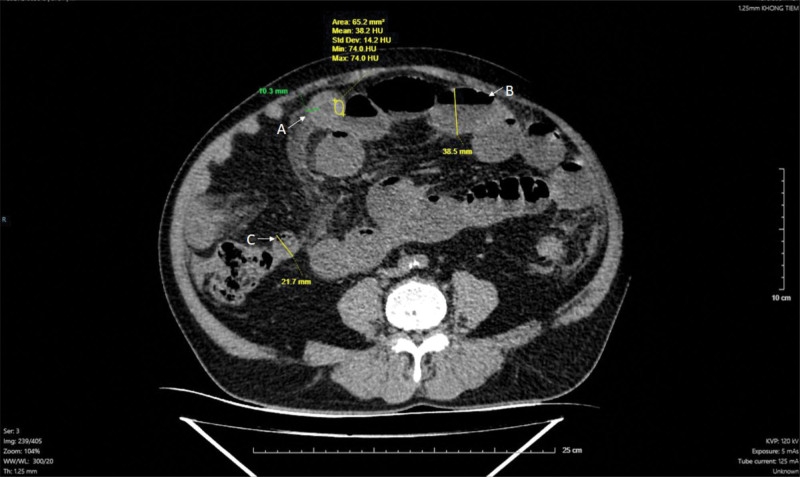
Non-contrast abdominal CT showing intramural small-bowel hematoma. (A) Segmental circumferential bowel wall thickening (approximately 10–12 mm) with increased intramural attenuation (mean ~38 HU, with focal values up to 70 HU), consistent with intramural hematoma. The lesion causes luminal narrowing and demonstrates a characteristic “target sign” appearance. (B) Proximal bowel dilatation with air–fluid levels upstream of the narrowed segment. (C) Collapsed distal bowel segment beyond the site of luminal narrowing. CT = computed tomography.
